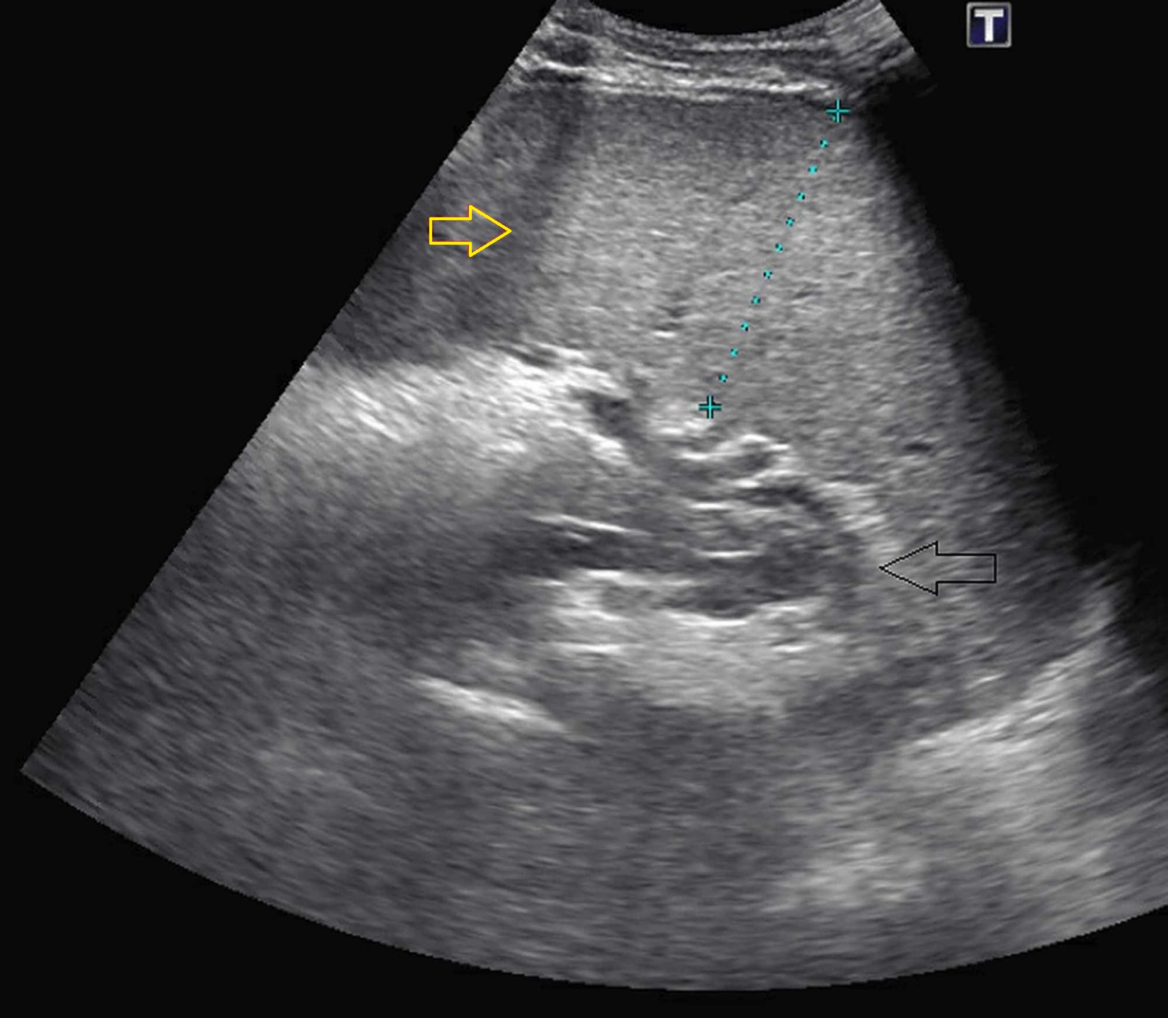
Patient's right upper quadrant ultrasound showing splenomegaly (yellow arrow) Yellow arrow: enlarged spleen; black arrow: stomach
